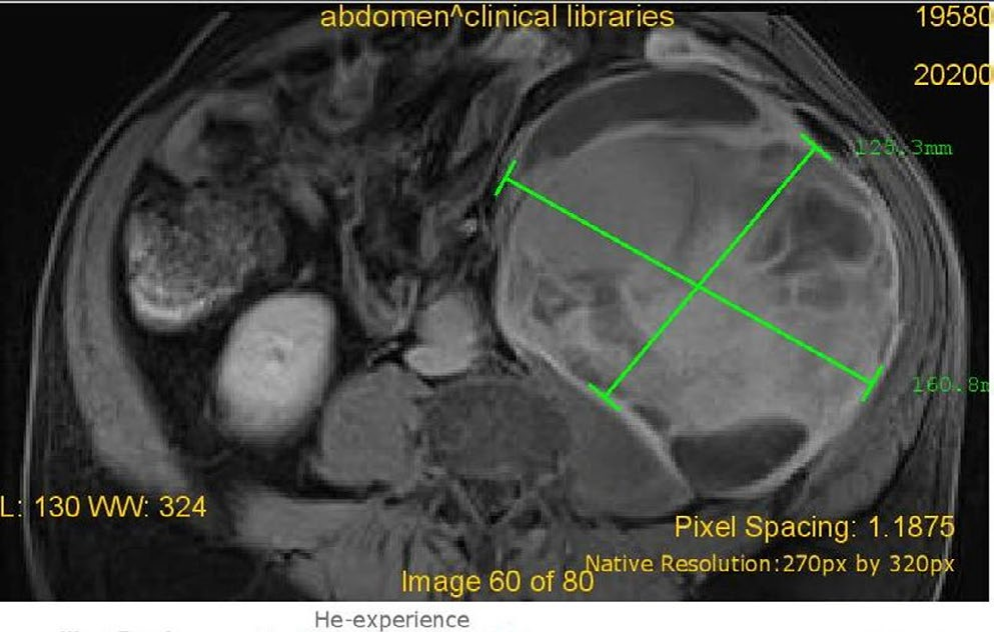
Sixty‐two‐year‐old man with recurrent retroperitoneal liposarcoma; MRI 2 months after the CT scan. The mass has increased in size about 4 cm
